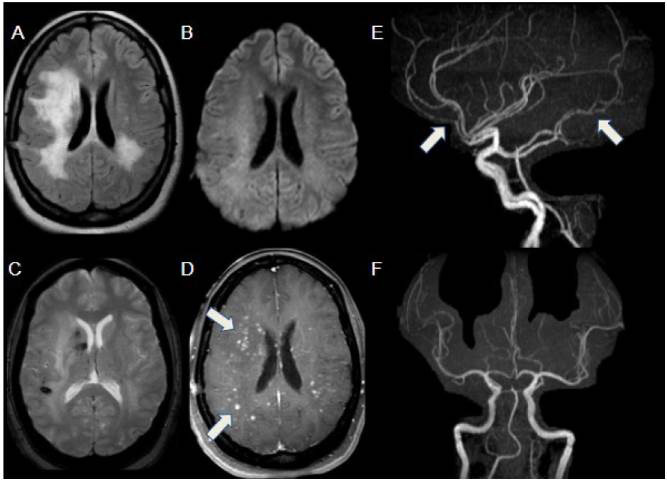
Primary angiitis of the central nervous system, in 17-year-old female with progressive unexplained neurological deficits. Axial FLAIR image (A) shows an extensive area of abnormal signal in the right frontoparietal white matter and left peritrigonal white matter, without restricted diffusion (B). SWI (C) depicts foci of magnetic susceptibility in the right caudate and subcortical white matter, compatible with microhemorrhages. After administration of gadolinium (D), multiple bilateral parenchymal nodular foci of enhancement are evident in the areas of abnormal FLAIR signal, with perivascular distribution (arrows). MRA images (E and F) show irregularities in multiple distal arterial branches (arrows), consistent with vasculitis.
